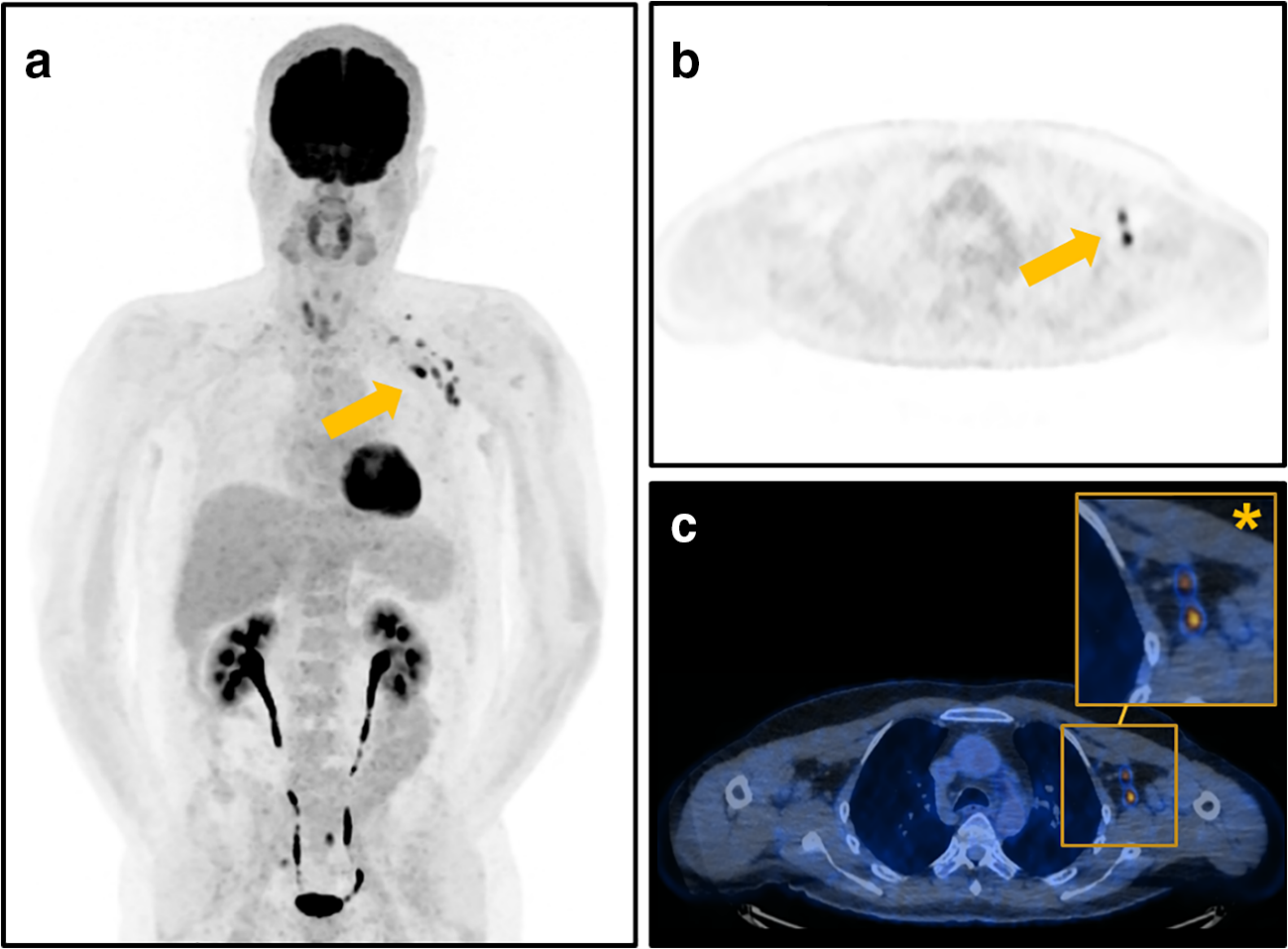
Representative images of a 53-year-old patient who underwent [^18^F]FDG PET/CT for restaging after resection of a melanoma of the left arm with FDG-avid axillary lymphadenopathy (arrows) after COVID-19 vaccination. The patient was scanned 3 days after the second vaccination with Moderna administered on the left side with SUV_max_ 11.6 of axillary lymph nodes. a Maximum intensity projection showing FDG-avid axillary lymph nodes, (b) axial PET image, and (c) fused PET/CT images with magnified image of the left axilla (asterisk). Due to the site of the primary lesion on the left arm and the FDG-avid lymph nodes, the patient was scheduled earlier for FDG PET/CT follow-up
